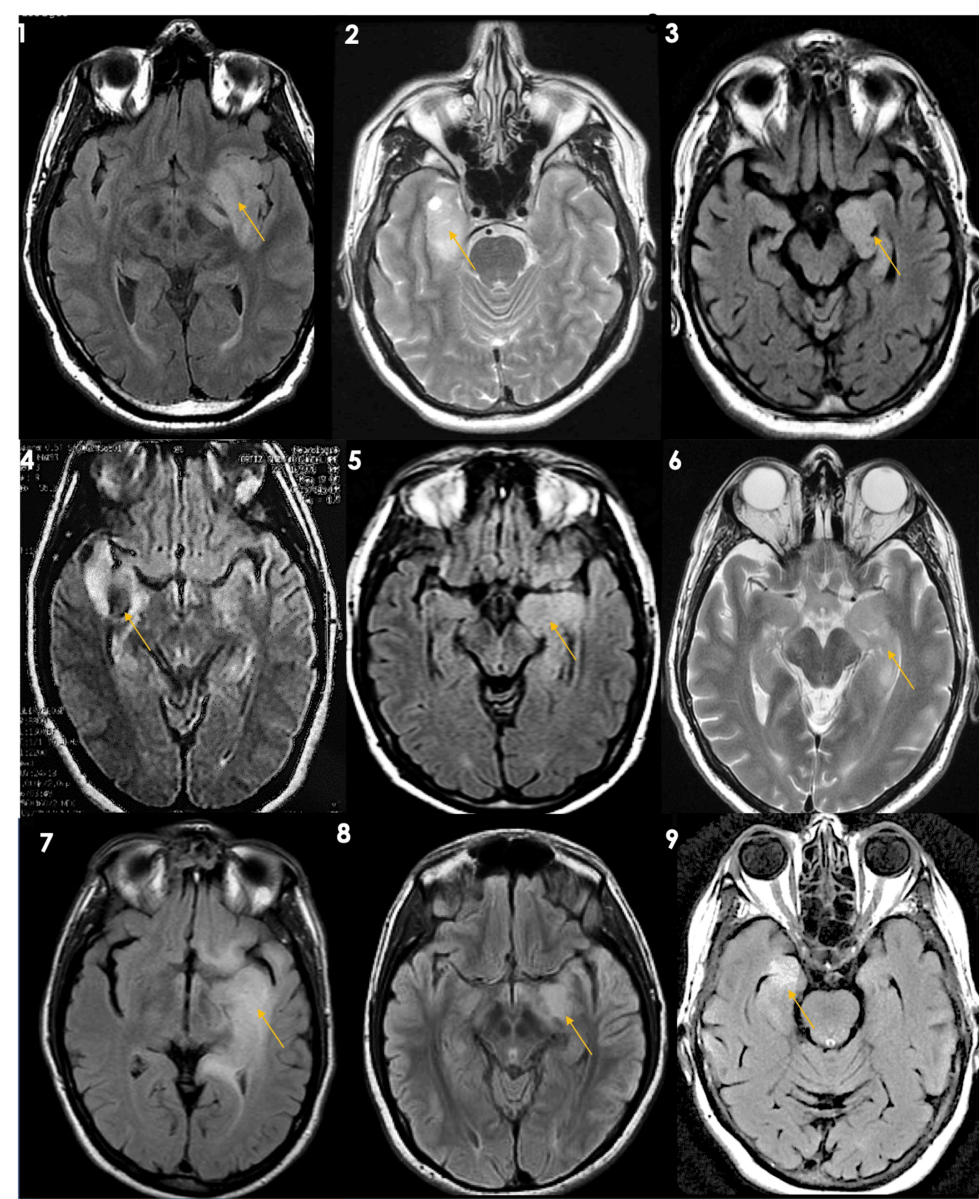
MRI series of the nine patients MRI: magnetic resonance imaging MRI series of the nine patients from 1 to 9, respectively, presenting unilateral hyperintense diffuse lesions pointed in yellow
